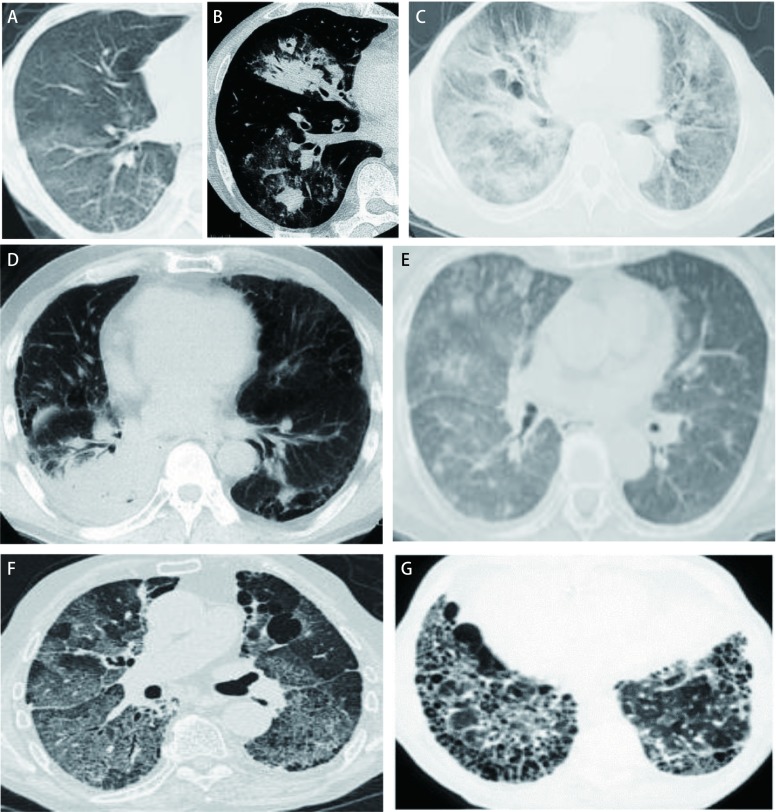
EGFR-TKI相关性ILD胸部CT特征。A：磨玻璃样改变；B：牵拉性细支气管扩张；C：急性间质性肺炎样改变；D：机化性肺炎样改变；E：嗜酸粒细胞性肺炎样改变；F：网织状改变；G：蜂窝状改变。 CT Features of EGFR-TKI-induced interstitial lung disease
